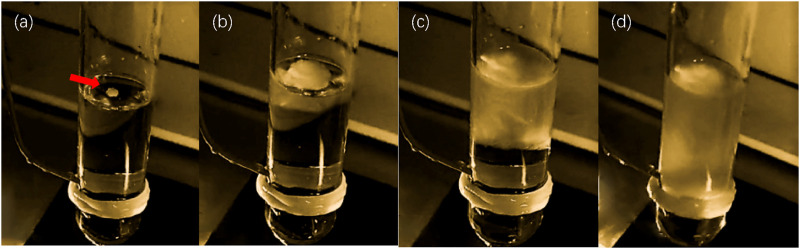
The effect of ultrasonic ice planting and nucleation. (a) Image of ice crystal growth after ultrasonic ice seeding for 1 s; (b) Image of ice crystal growth after ultrasonic ice seeding for 2 s; (c) Image of ice crystal growth after ultrasonic ice seeding for 4 s; (d) Image of ice crystal growth after ultrasonic ice seeding for 8 s.
